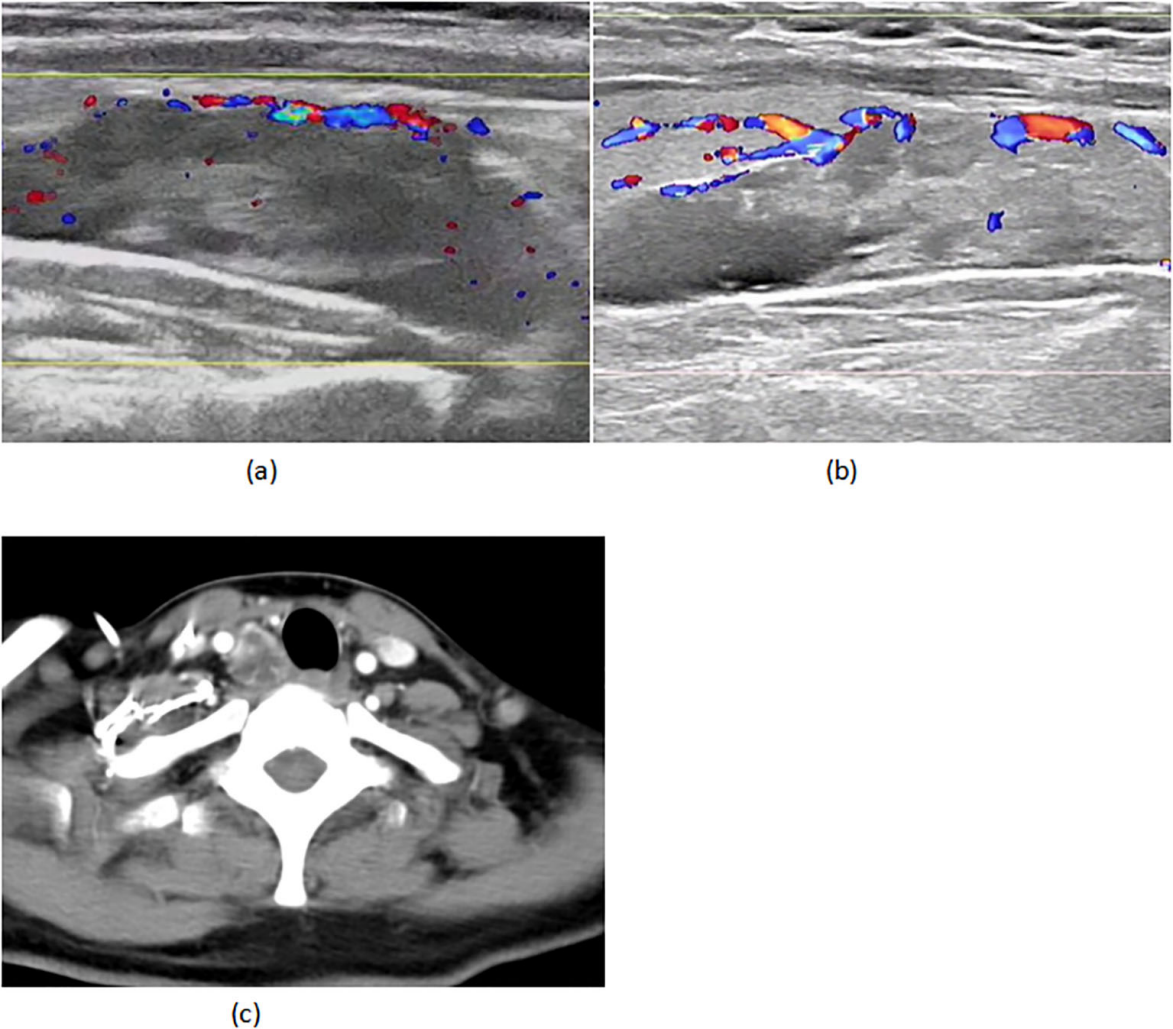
(A) 02–29 parathyroid ultrasound (B) 05-18 parathyroid ultrasound (C) 05–18 CT of the neck.
